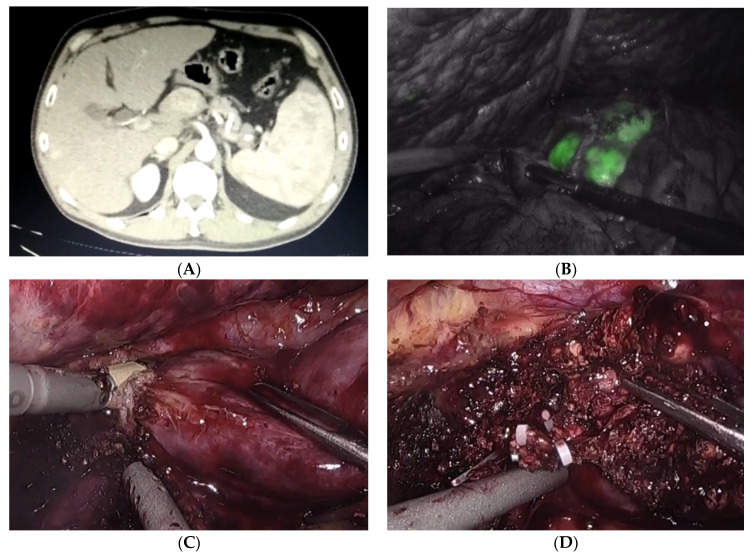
Laparoscopic resection of caudate lobe for hepatocellular carcinoma. (A) CT scan with arterial wash-in. (B) ICG enhancement of the lesion, assuring negative resection margins. (C) Parenchymal transection. (D) Securing spigelian vessels.
